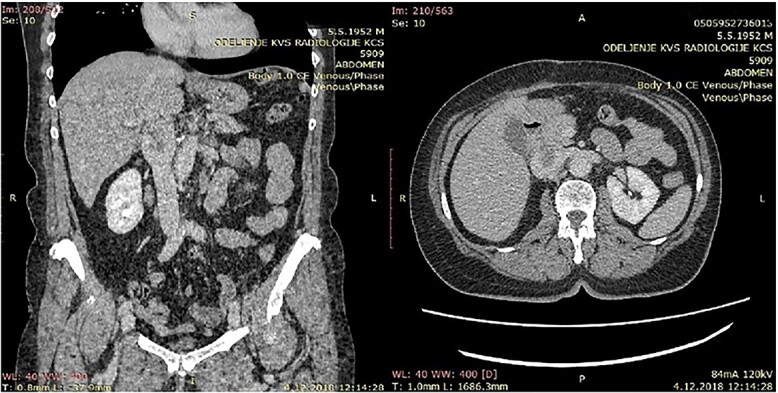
CT of the abdomen that shows a retroperitoneal tumor mass that involves IVC above the renal and under the liver veins.
